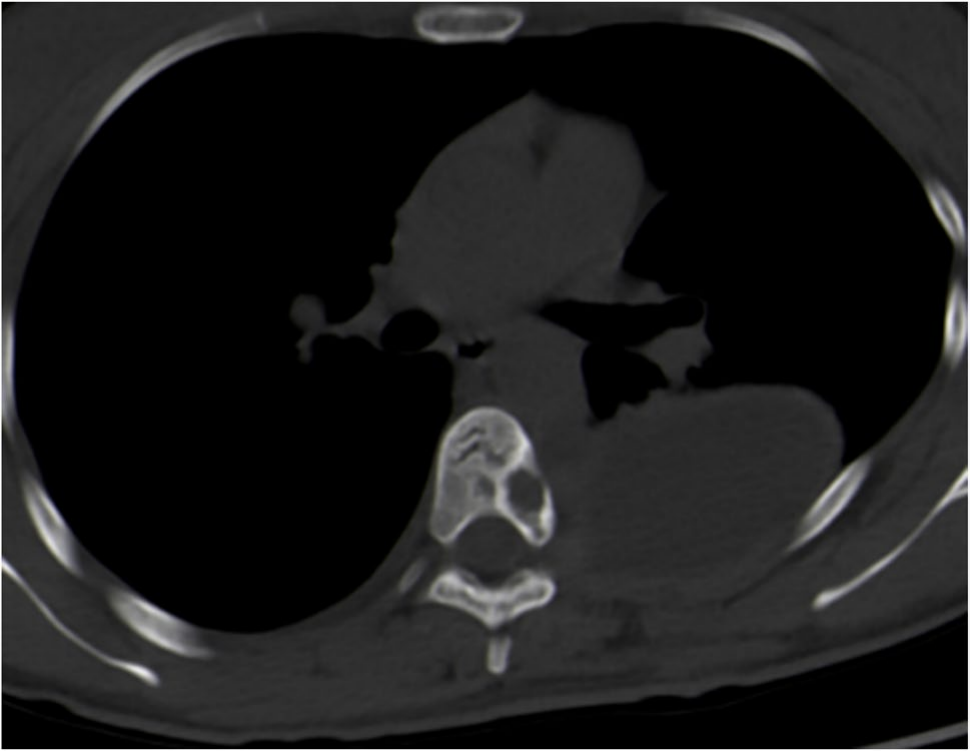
Plain CT scan showed cystic and expansive bone destruction of the 9th thoracic vertebrae with hydatid sac in the left soft tissue
